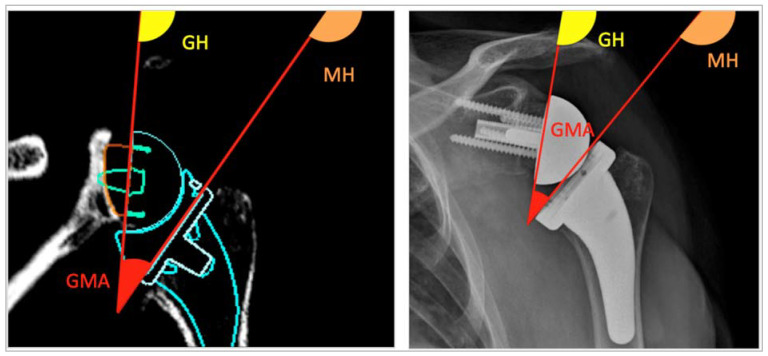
Angle measurements on planning 2D-CT coronal view (left) and post-operative anteroposterior X-ray view (right). Glenometaphyseal angle (GMA) in red, metaphyseal horizontal line angle (MH) in orange, and glenoid horizontal line angle (GH) in yellow.
